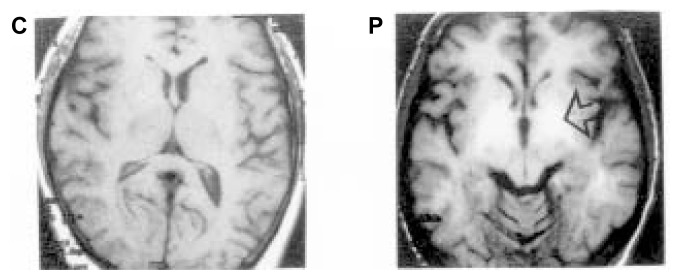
Magnetic resonance imaging (MRI) of a healthy control subject (C) and an alcoholic cirrhotic patient of the same age (P). In the alcoholic patient, abnormally intense signals (arrow) are detected on both sides of the brain in a region called the globus pallidus. This phenomenon has been attributed to deposits of manganese in this brain area. SOURCE: Lockwood et al. 1997.
